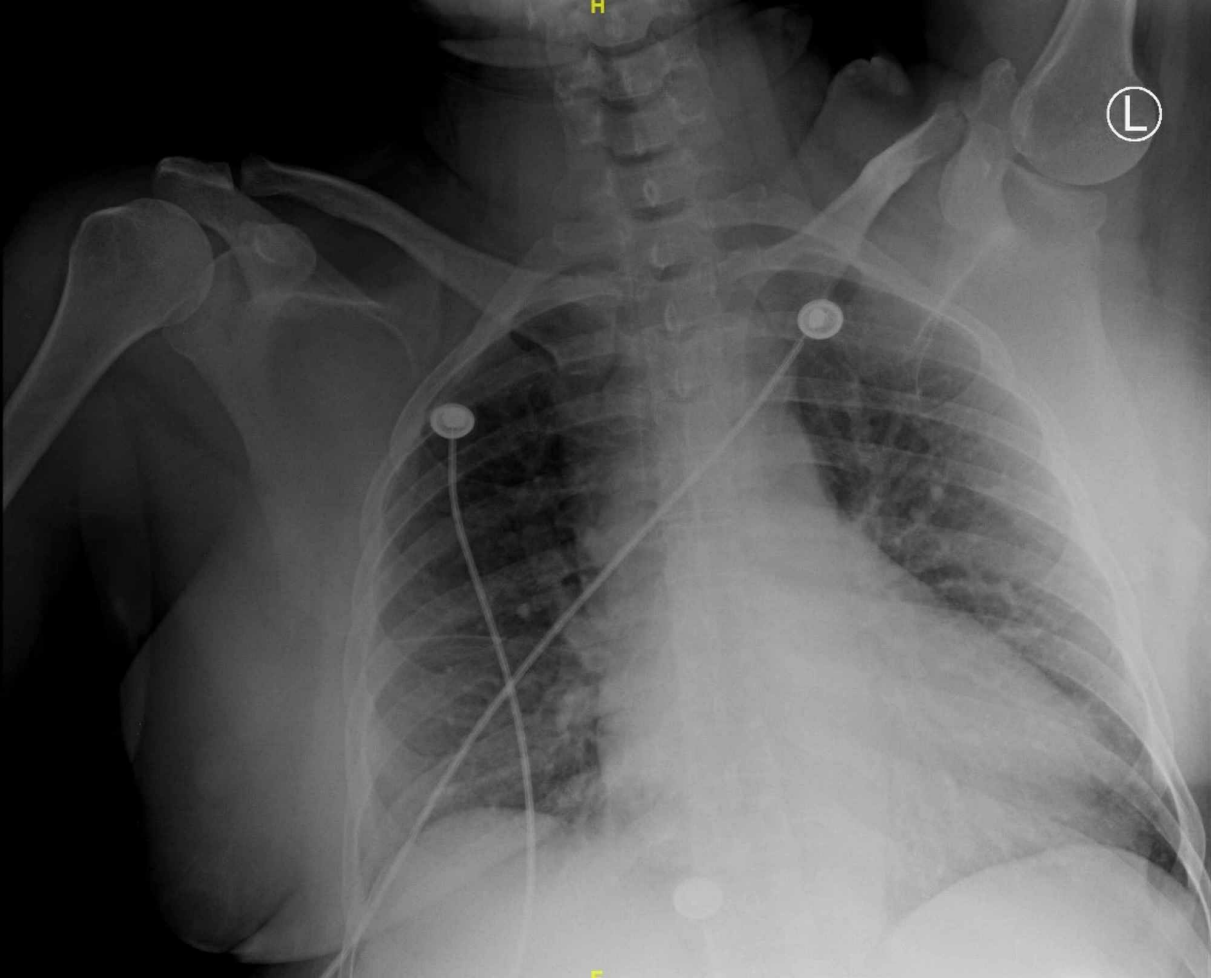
Anteroposterior X-Ray of the Chest Demonstrating clear bilateral lung fields with overlying breast tissue opacities and a normal-sized cardiac silhouette.
